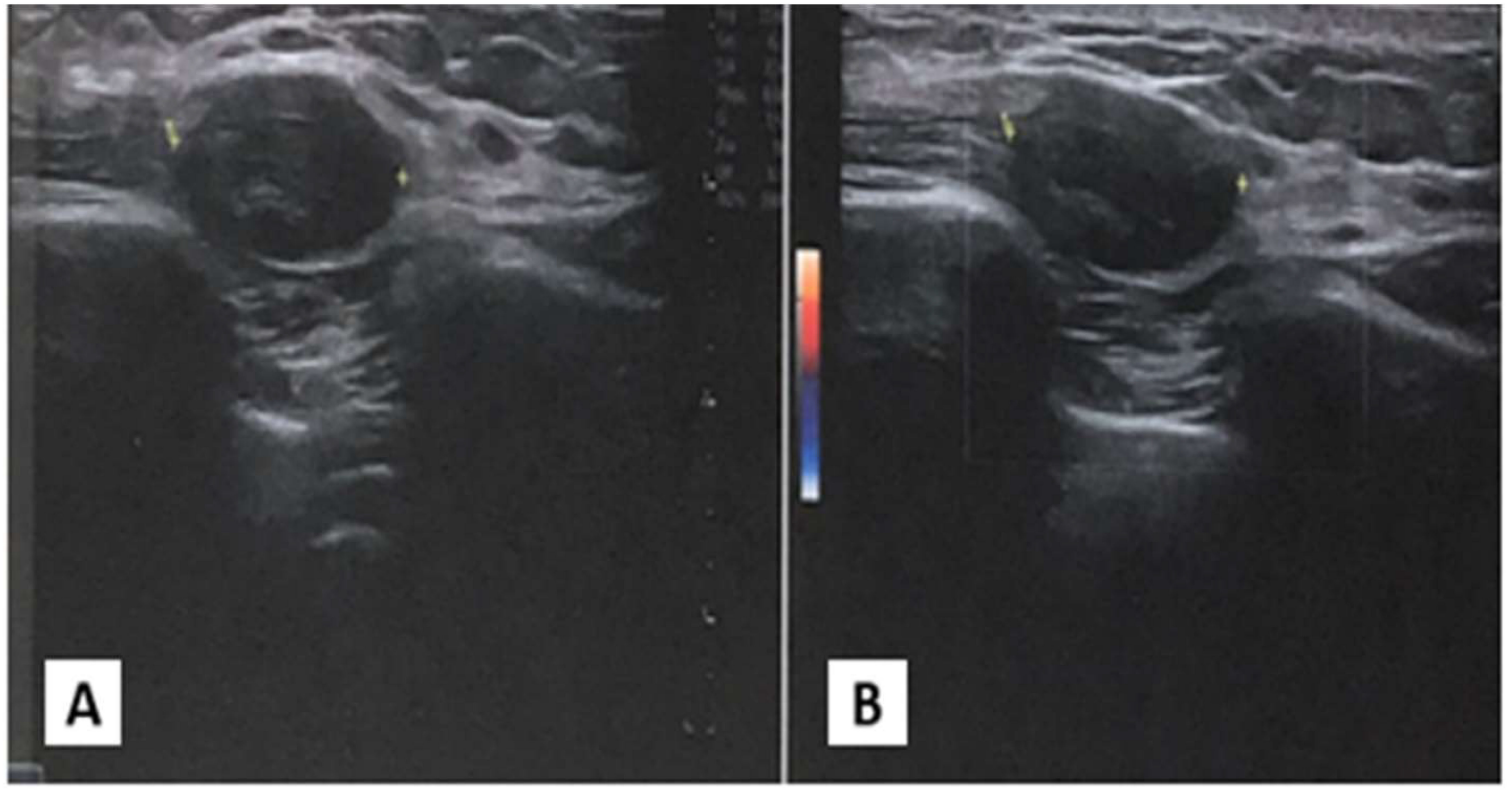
Ultrasonography of chest wall. (A) Ipoechoic soprafascial nodular mass with 2 cm x 2xm of diameter. (B) Absence of signal to Color Doppler examination.
